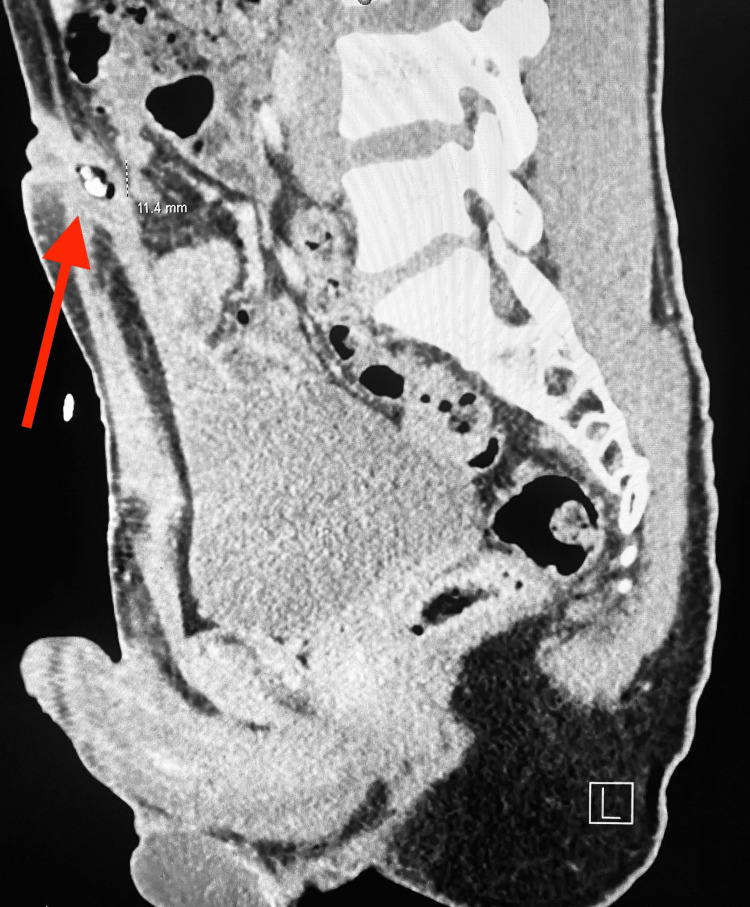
CT of the abdomen and pelvis Computed tomography (CT) images revealed skin thickening in the umbilicus with a subcutaneous central area of mixed attenuation approximately 1.7 cm deep to the skin surface measuring 1.3 x 1.1 x 1.1 cm and a smaller cutaneous collection with similar attenuation measures 0.6 x 0.5 x 0.7 cm, both containing calcified material.
